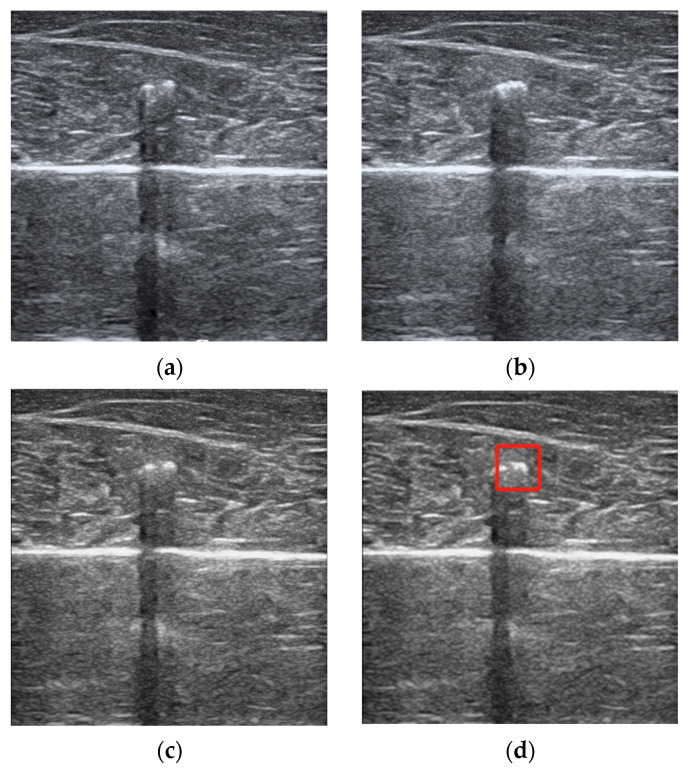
(a) ultrasound image before heating; (b) ultrasound image after heating; (c) image fused along the time dimension; (d) image after mean filtering.
